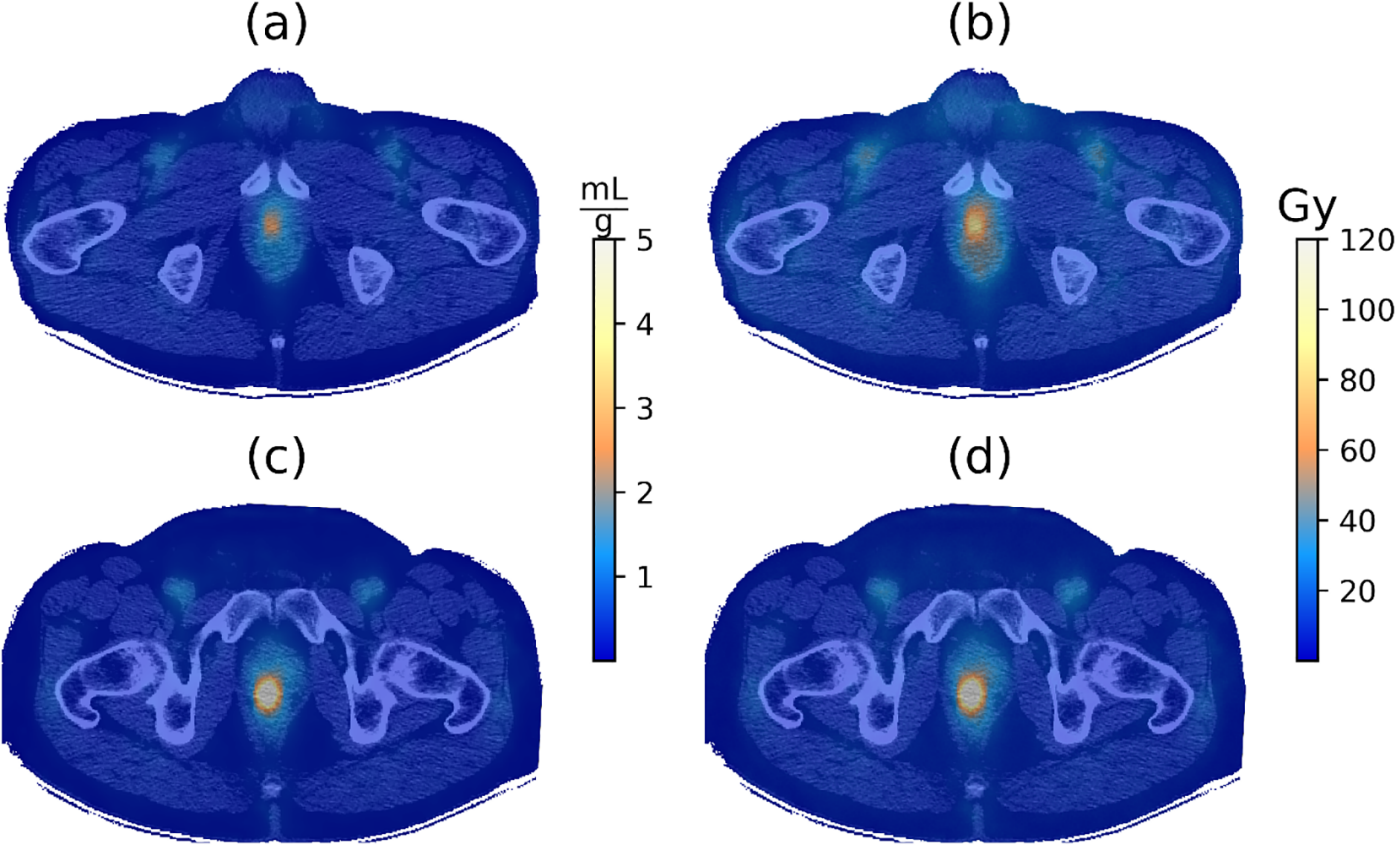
(a) Logan distribution volume (LDV) and (b) absorbed dose maps overlaid on the corresponding CT image for patient IGPC-02-029. (c) LDV and (d) absorbed dose maps overlaid on the corresponding CT image for patient IGPC-02-026. The BED was calculated using the parameters from Table 3.
